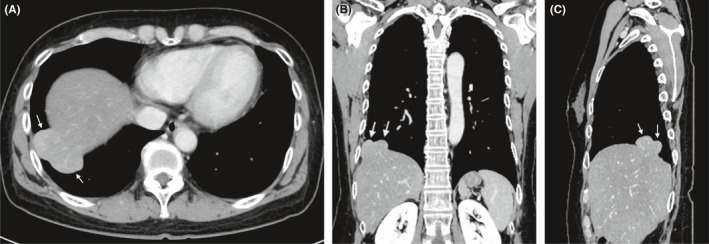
Thoracoabdominal‐enhanced computed tomography revealed smooth‐bordered masses located next to the liver. (A) Axial view, (B) coronal view, (C) sagittal view. Arrows indicate liver herniations
